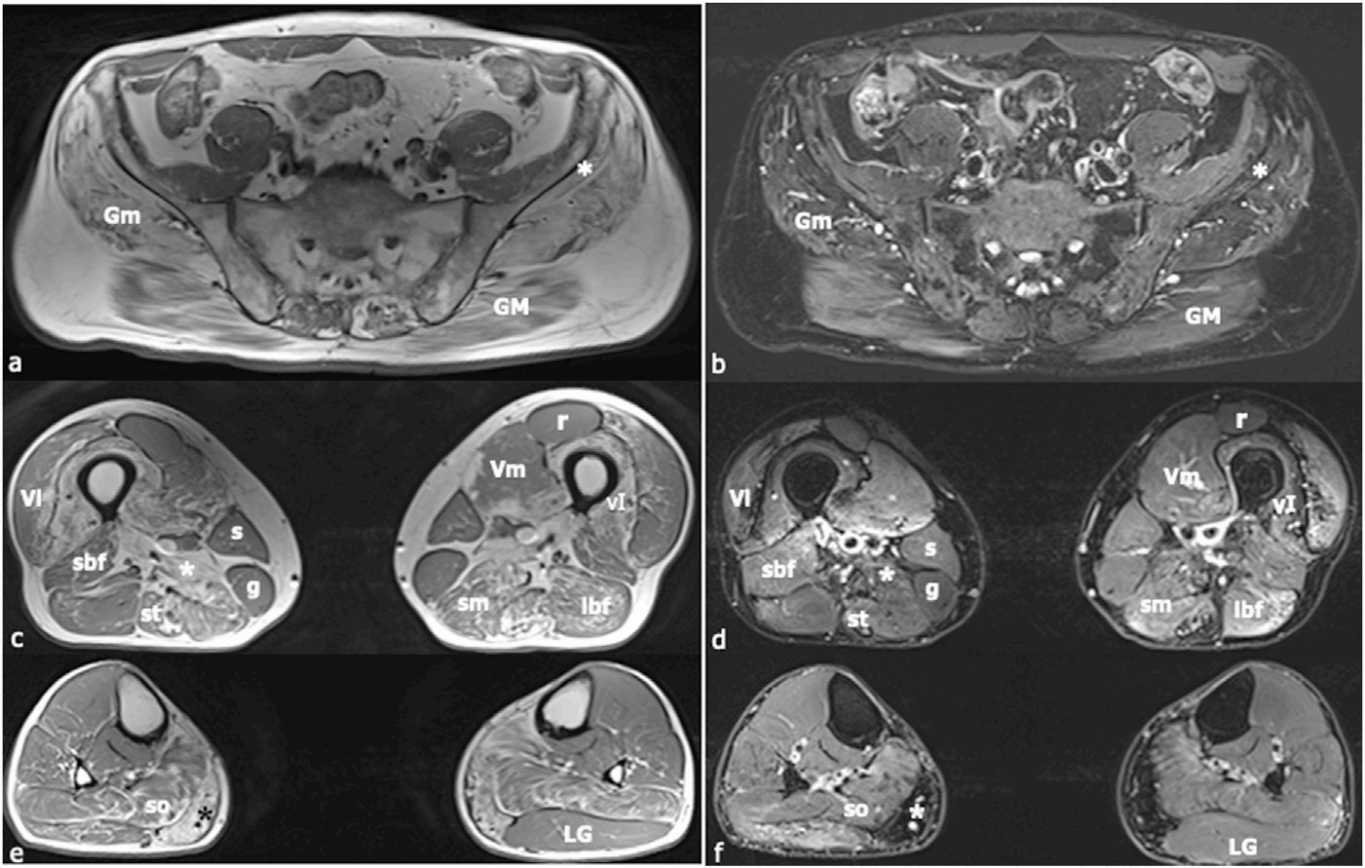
Muscle Magnetic Resonance Imaging (MRI). In (A), (C), and (E) axial, T1-weighted images of the lower limbs are shown, whilst in (B), (D), and (F) axial T2-weighted images with fat-saturation are displayed. Images (A) and (B) were acquired at the level of the first sacral vertebra, images (C) and (D) at the midportion of the thigh, and images (E) and (F) at the proximal third of the leg. Increased T1-signal related to fibrous and fat infiltration was observed in the gluteus maximus (*GM*), gluteus medius (*Gm*), gluteus minimus [white asterisk in (A), (B)], semimebranosus (*sm*), semitendinosus (*st*), adductor magnus [white asterisk in (C), (D)], vastus intermedius (*vI*), medial gastrocnemius [black asterisk in (E), white asterisk in (F)], and soleus (*so*) muscles. A mild signal intensity increase in the T2-weighted sequence was found in the short head of the biceps femoris (*sbf*), long head of the biceps femoris (*lbf*), semimembranosus (*sm*), and semitendinosus (*st*) muscles, corresponding to muscle necrosis and consequent inflammatory response. The sartorius (*s*), gracilis (*g*), and lateral gastrocnemius (*LG*) muscles did not present any morphological changes. Muscle alteration pattern is consistent with the findings previously described in autosomal dominant calpainopathies. *Vl* = vastus lateralis; *Vm* = vastus medialis; *r* = rectus femoris.
